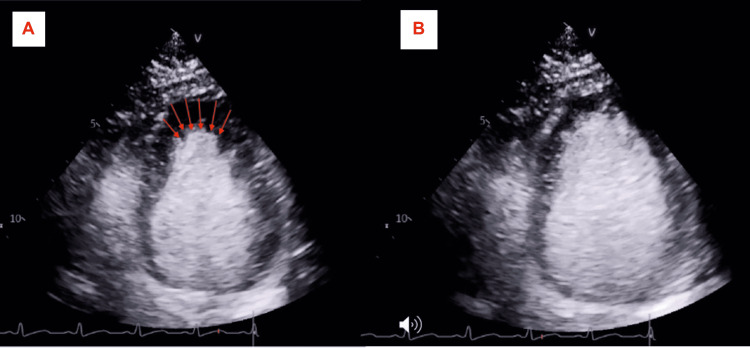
Apical four-chamber view on TTE showing basal akinesis with preserved apical contraction (A, B).
